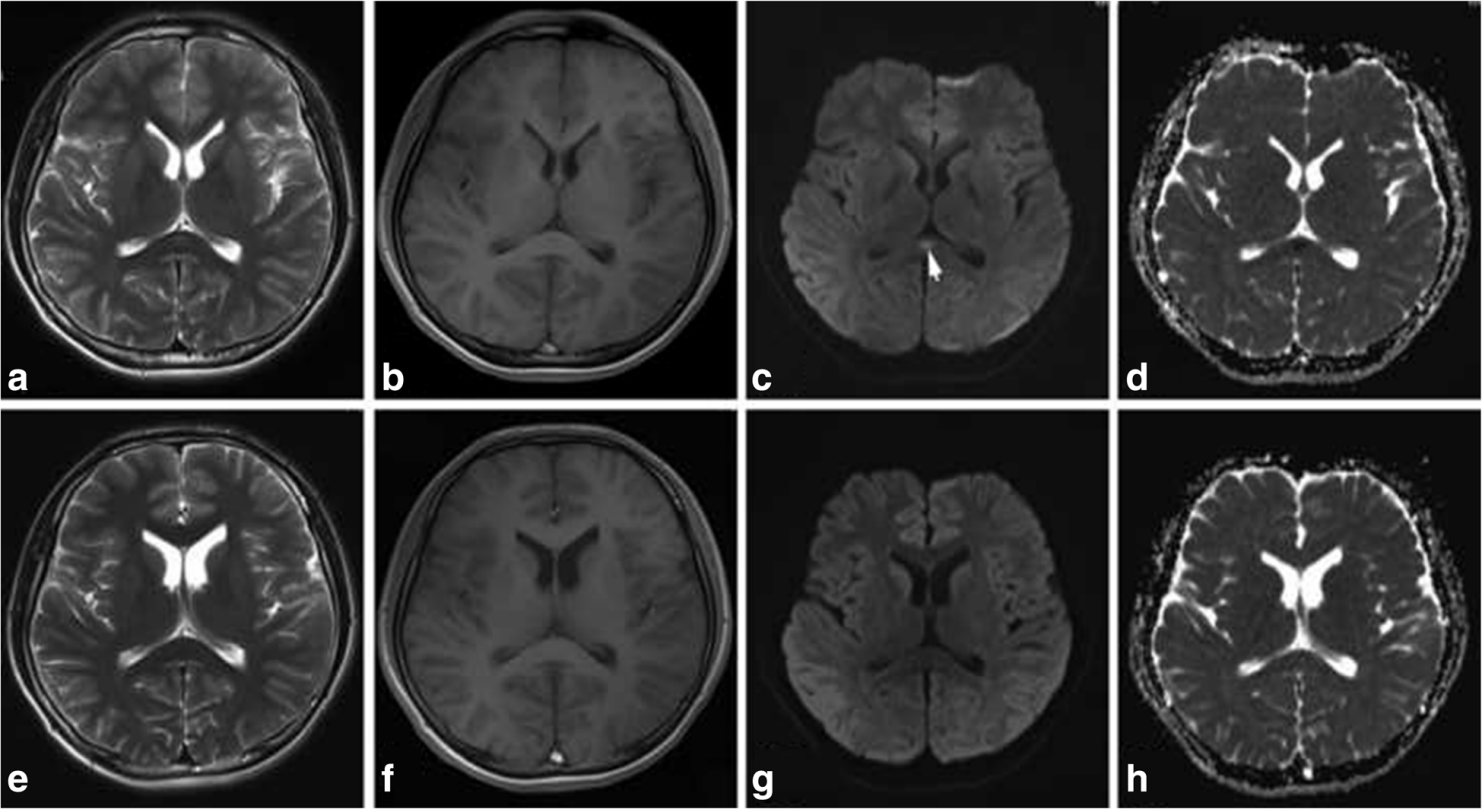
The cerebral MRI features of case 7. MRI showed an isolated SCC lesion with slight hyperintense on T2WI (a), slight hypointense on T1WI (b), hyperintense on DWI (c, *arrow*), and obvious decreased ADC values (d). At 32 days after first MRI, second MRI showed abnormal signals of the SCC completely disappearing on T2WI (e), T1WI (f), DWI (g) and ADC (h)
